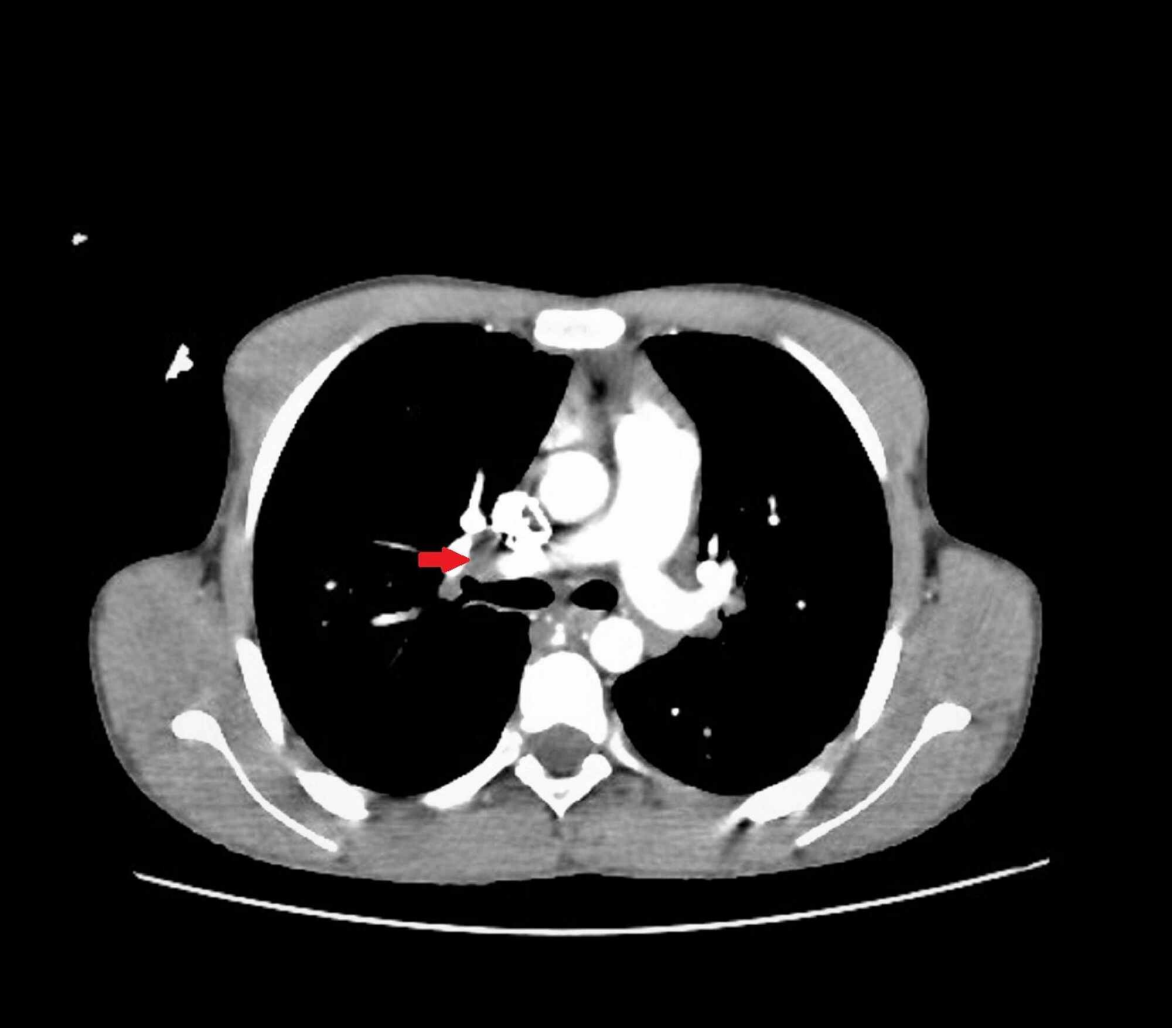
Chest CT scan showing a lobar inferior pulmonary embolism (red arrow).
